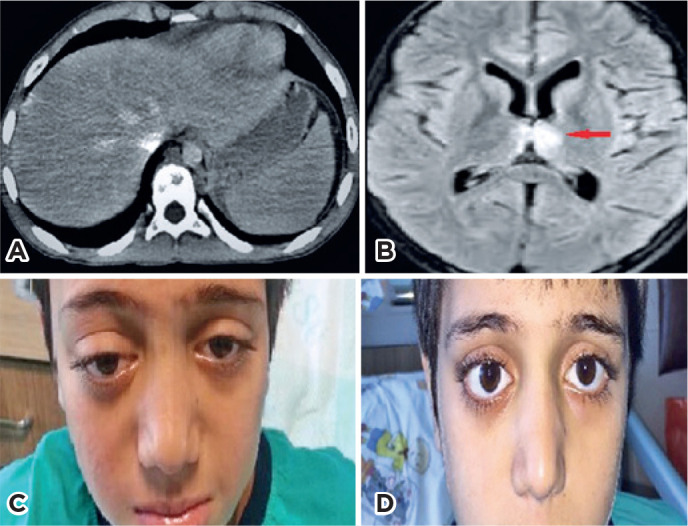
Case 14: (A) Abdominal computed tomography image showing lytic lesions on
